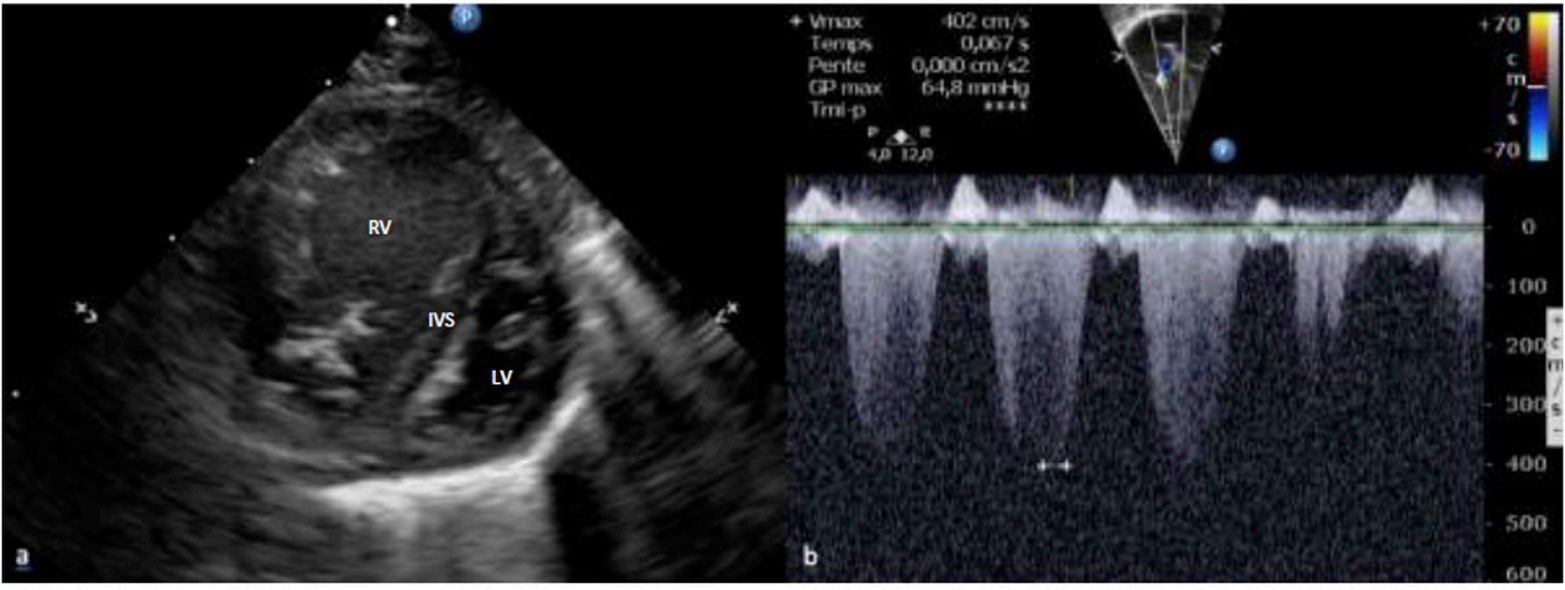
Supra-systemic pulmonary hypertension was assessed on echocardiography. Parasternal short axis ventricles (A): Bowing of the interventricular septum into the left ventricle. Apical 4 chamber (B): Tricuspid regurgitation blood flow velocities higher than 4 m.s^−1^. RV: Right Ventricle; LV: Left Ventricle; IVS: Interventricular Septum.
